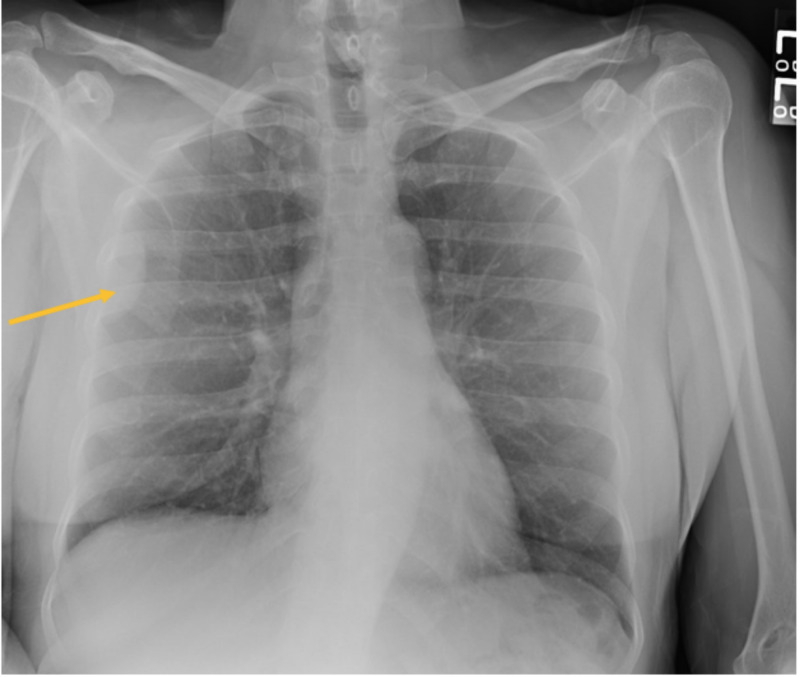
Chest X-ray showing solitary fibrous tumor.
